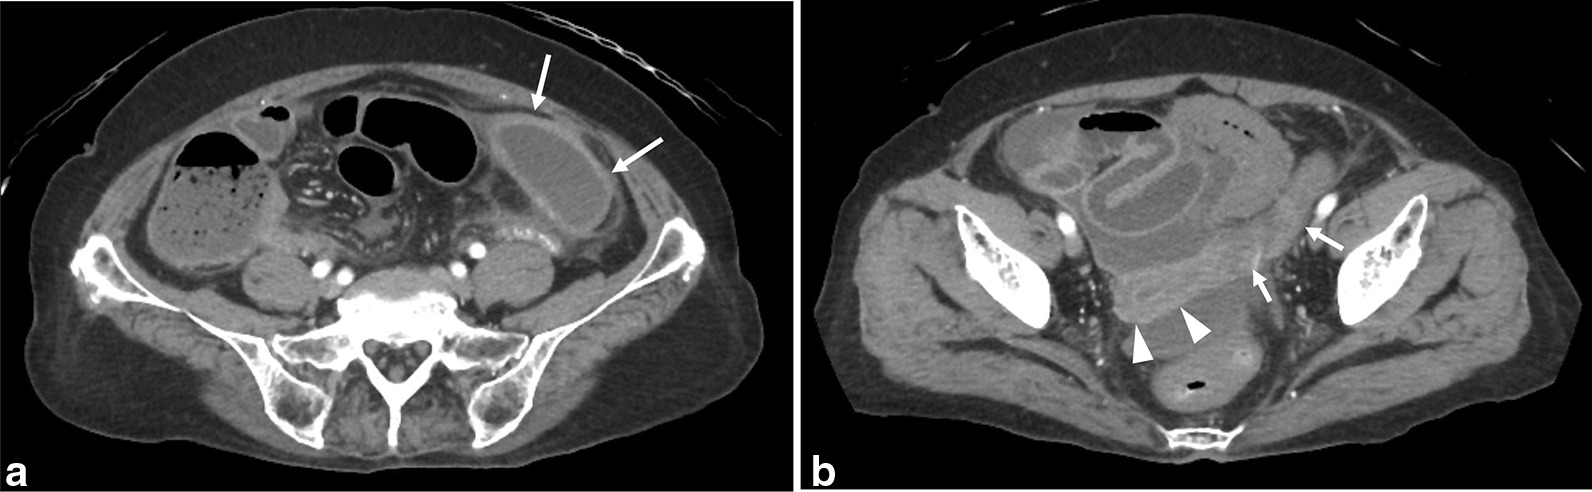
Enhanced abdominal computed tomography demonstrates a cystic mass of 64 × 30 mm with an equally enhanced wall in the left pelvic cavity (panel a, arrows). The cystic mass is continuous with the left edge of the uterus (panel b, Arrowheads show the uterus, and arrows show a cord structure connected to the mass)
